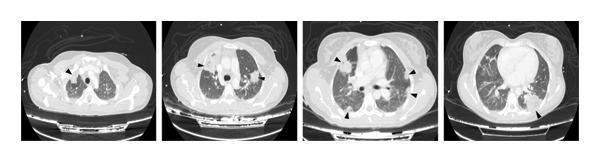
CT‐scan (axial view) in which multiple bilateral lung consolidations can be seen (black arrows).
